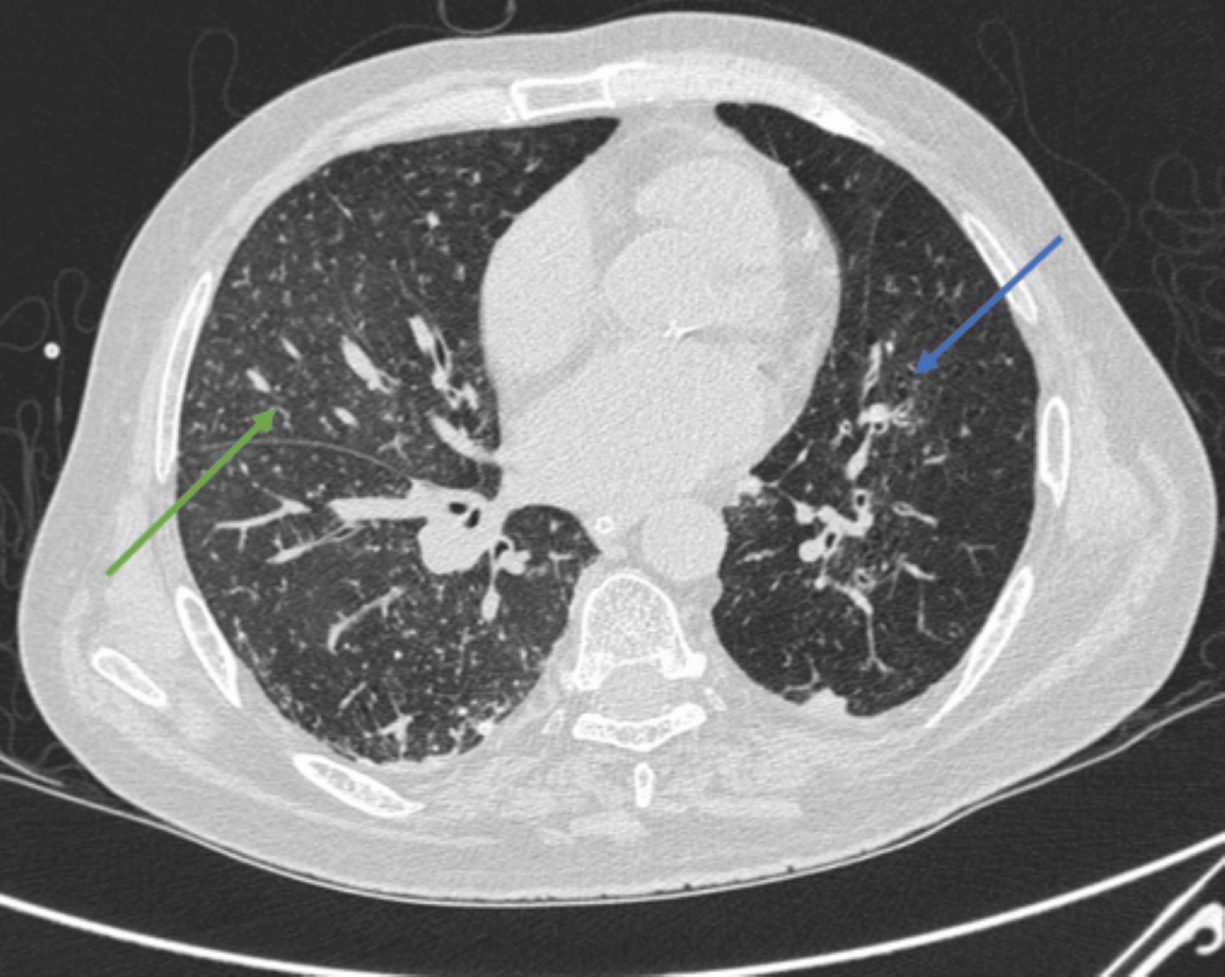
CT scan of the chest showing centrilobular emphysema (blue arrow) and mosaic attenuation with centrilobular nodules (green arrow) suggestive of interstitial disease
